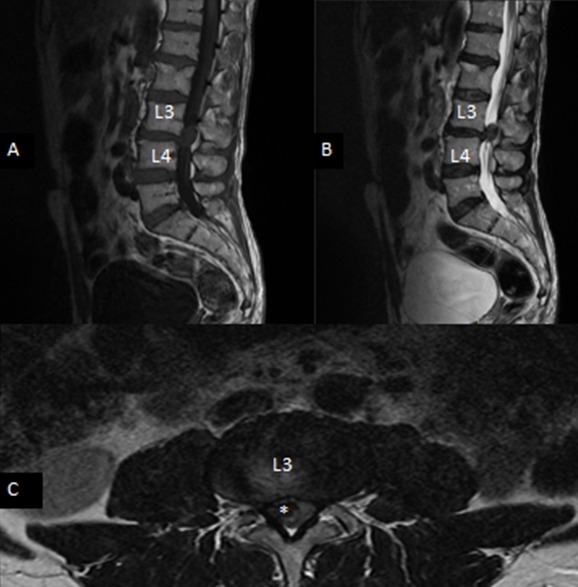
Sagittal T1 (A), sagittal T2 (B), and axial (C) T1-weighted MR images revealing a right posterolateral epidural mass (star) that compressed the dural sac at the L3-4 level
